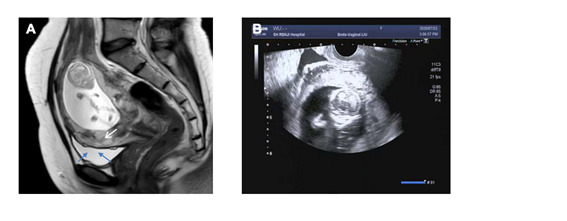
A: MRI showing on the T2W uterine median sagittal plane the placenta completely covering the cervical internal orifice and being accrete to the muscular layer. Part of the placenta breaks through the muscular layer and protrudes into the bladder cavity like a tent (see black arrow). B: Ultrasound showing the presence of a live foetus with placenta previa and its partial uterine implantation.
